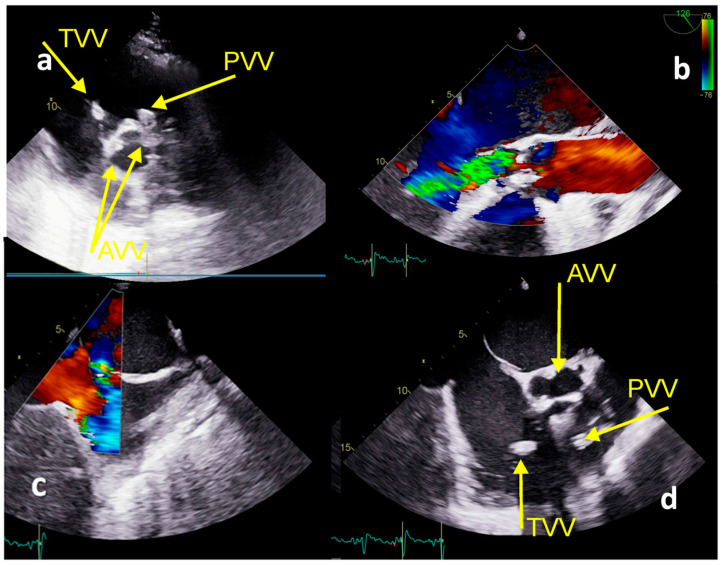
Echocardiography TTE and TEE imaging−Case 3. (a) TTE: TVV−vegetation at the tricuspid valve, PVV−vegetation at the pulmonary valve, AVV−vegetations at the aortic valve; (b) TOE−severe aortic regurgitation; (c) TOE−patent foramen ovale; (d) TOE−vegetations at the tricuspid, pulmonary, and aortic valves.
